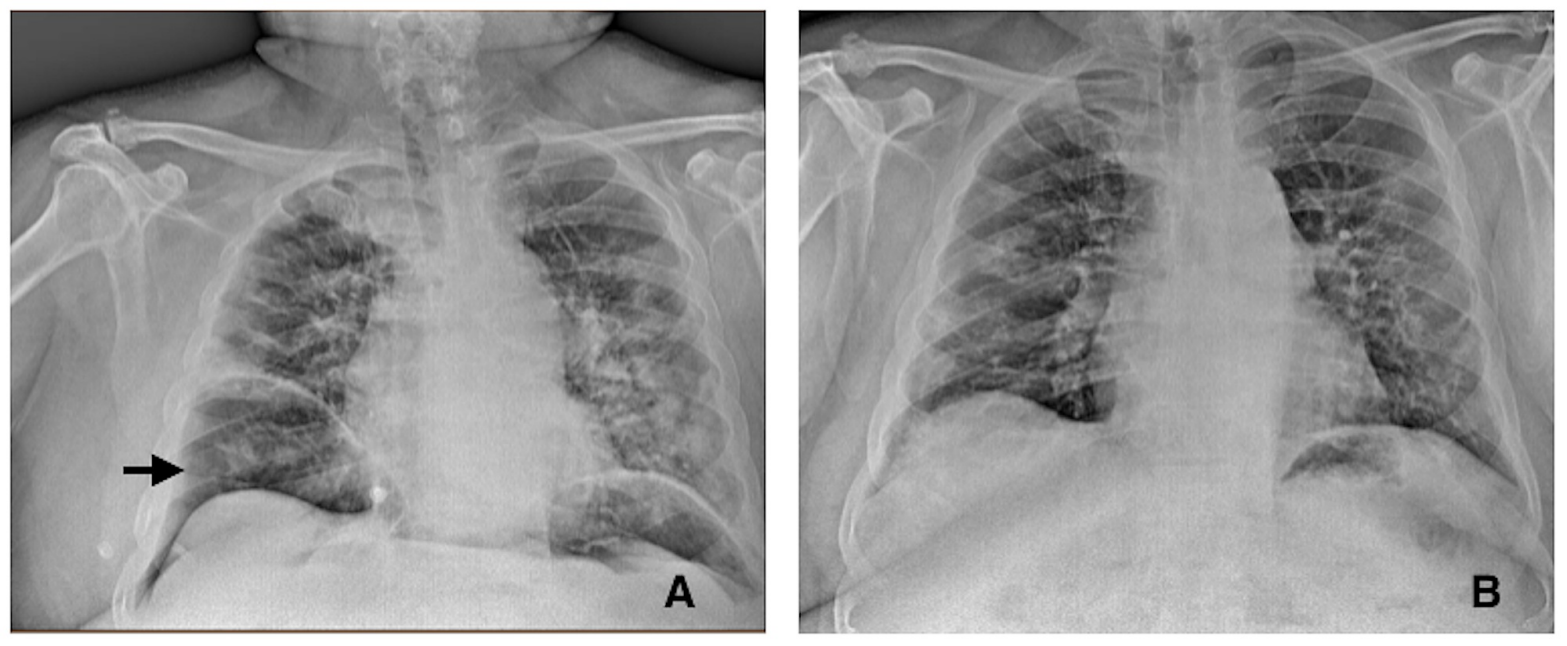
Chest radiograph. A: Index radiography with diffuse bilateral opacities, with subdiaphragmatic free air (arrow); B: Discharge radiography  with pneumoperitoneum resorption and diminish of the pulmonary inflammatory process.
